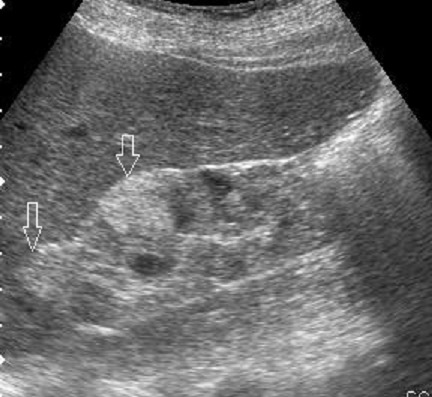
Ultrasound image shows renal cysts and angiomyolipomas seen as cystic and hyperechoic lesions in the kidneys (arrows)
